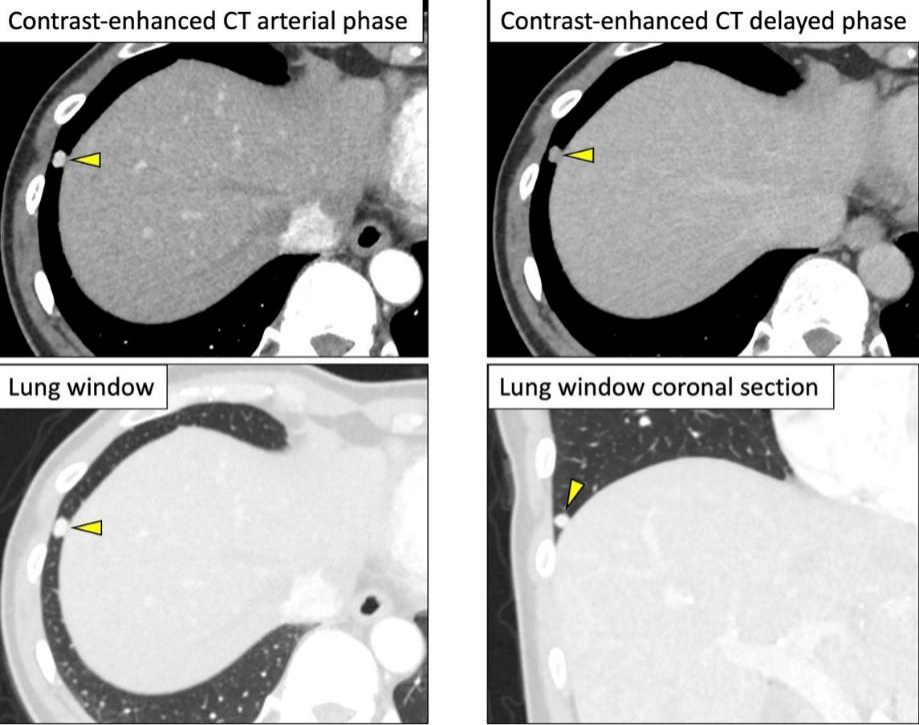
Contrast-enhanced CT showing a 0.7 × 0.5 × 0.7cm^3^ nodule in the right lung base that is strongly contrasted in the arterial phase and washed out in the delayed phase (▻)
